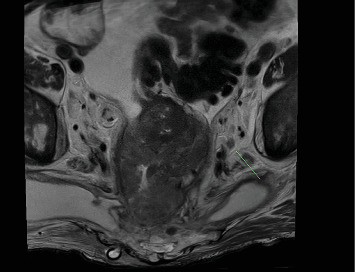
MRI Pelvis demonstrating the rectal tumor with complete invasion of the mesorectal plane, occupying the entirety of the muscularis propria and mesorectal fat.
